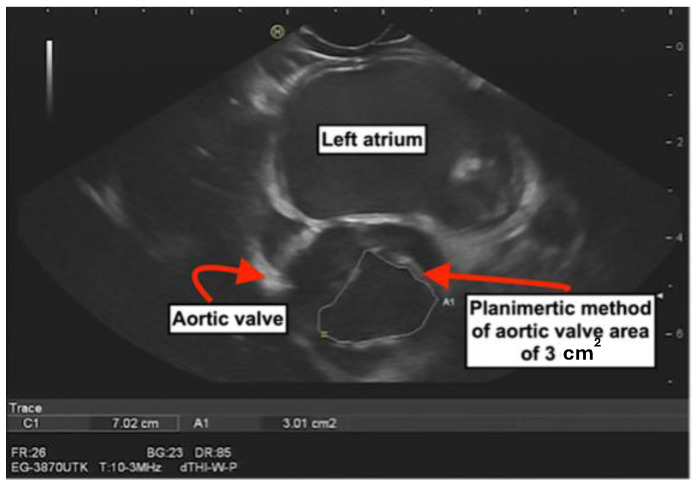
Endoscopic ultrasound demonstrated a planimetry method for the calculation of aortic valve area.
